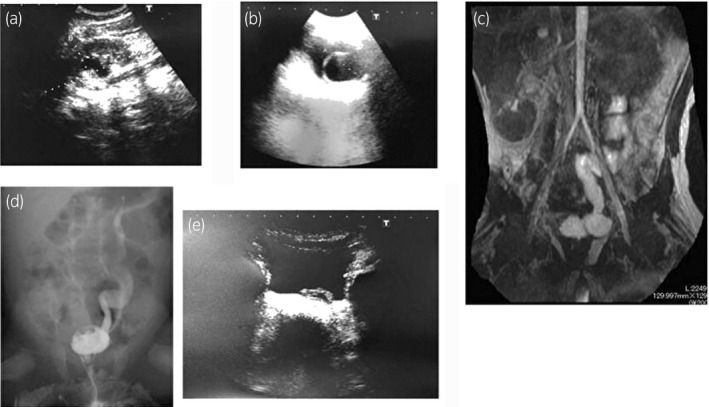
Patient 2. Ultrasonography of the left kidney (a), ultrasonography of the bladder showing an 11‐mm ureterocele (b), MRI showing ectopic ureterocele (c), VCUG showing Grade II VUR in the left superior kidney (d), and ultrasonography of the bladder after 2 months of conservative treatment showing reduction of the ureterocele (e).
